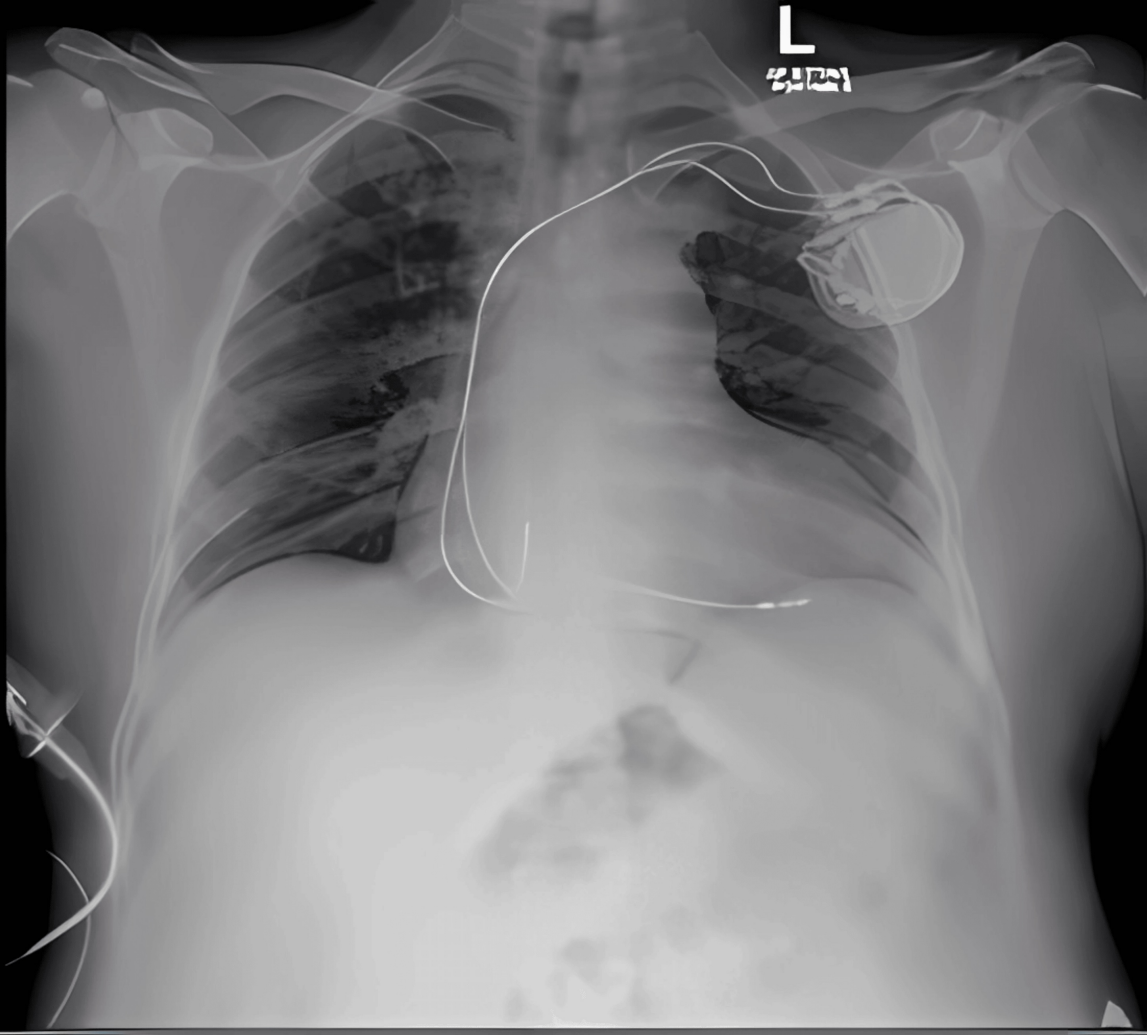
Serial chest radiograph demonstrating stable pacing lead position and no device‐related complications
